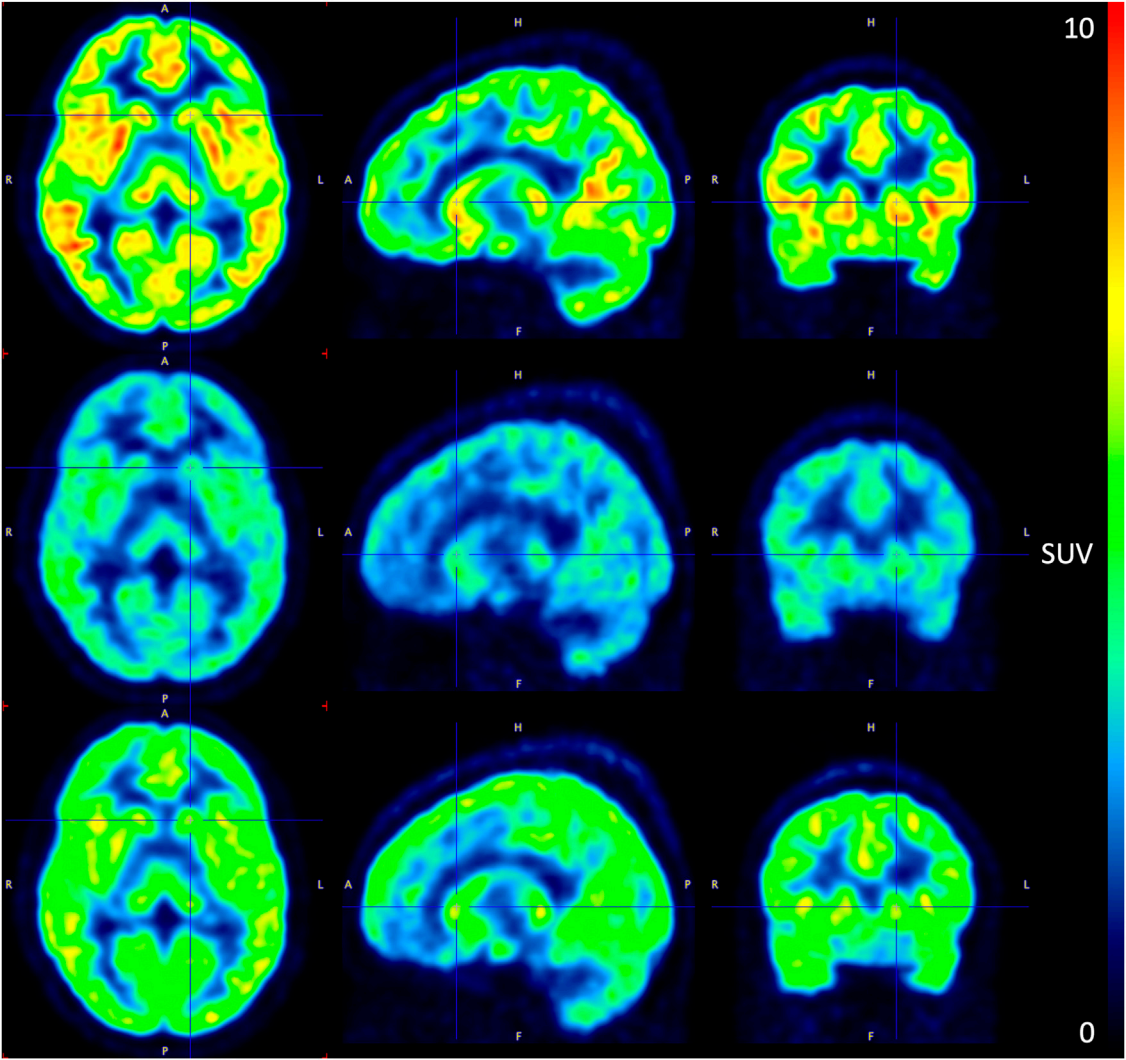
PET image of ^11^C-UBC-J binding during the pre-dose (top), T_max_ (mid), and 24 h post-dose (bottom) scan at the 10 mg SDI-118 dose level in a selected subject (left) axial plane (mid) sagittal plane (right) coronal plane. SUV: standardized uptake value.
